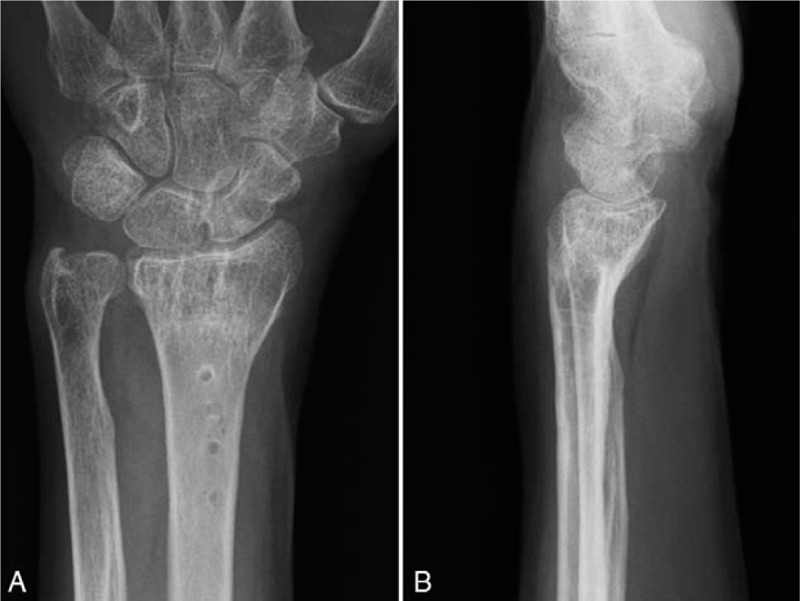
Radiographs at final follow-up 1 year postoperatively. (A) Anteroposterior and (B) lateral views show restored alignment of the distal radioulnar joint and corrected dorsal angulation of the distal radius.
